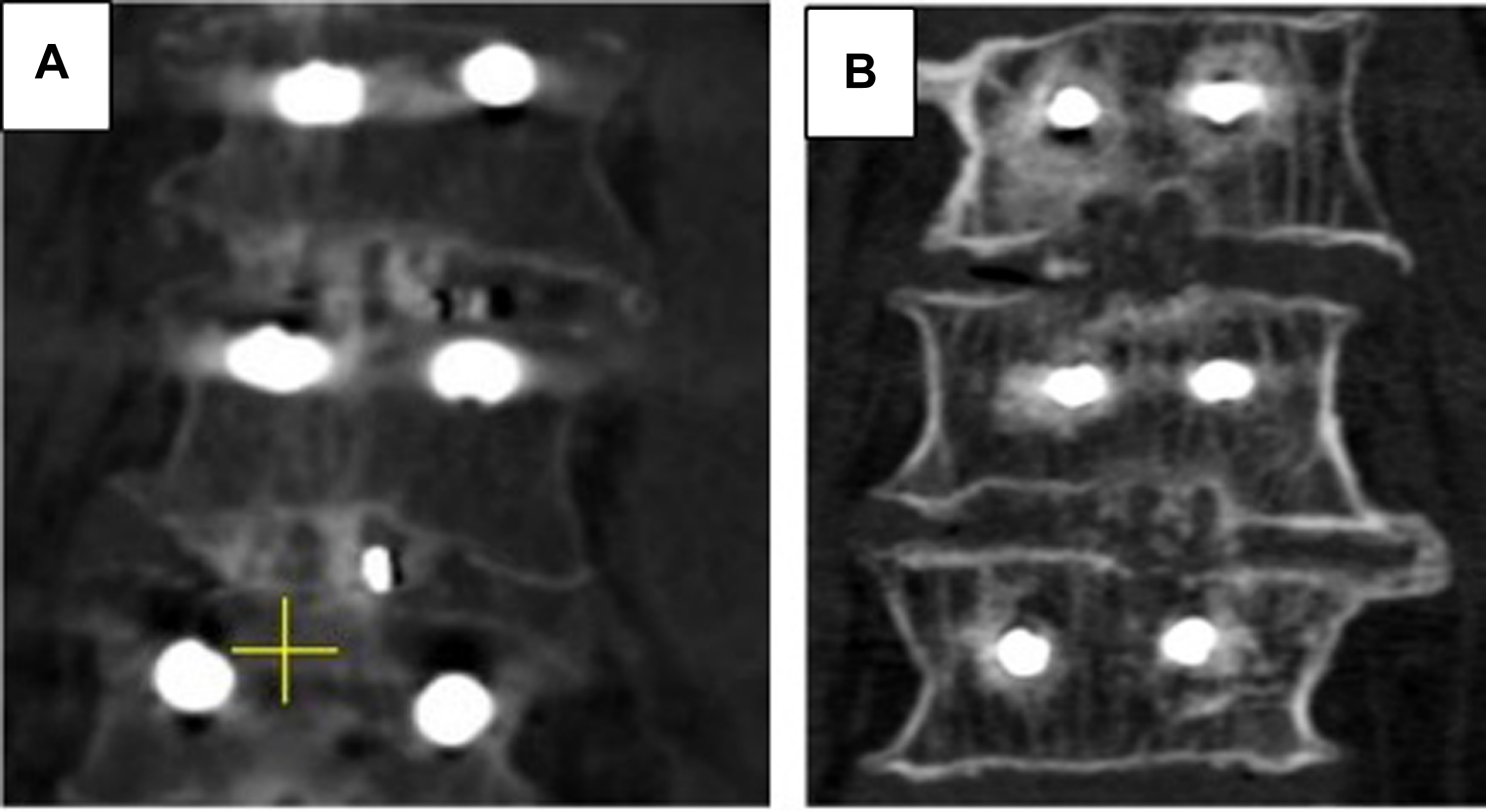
Grades of anterior fusion. (A) CT scan in coronal plane, 1 year after surgery, bipolar bridging was confirmed on both levels. (B) CT scan in coronal plane, 1 year after surgery, total non-union was detected on the upper operated and unipolar pseudoarthrosis – on the lower level.
